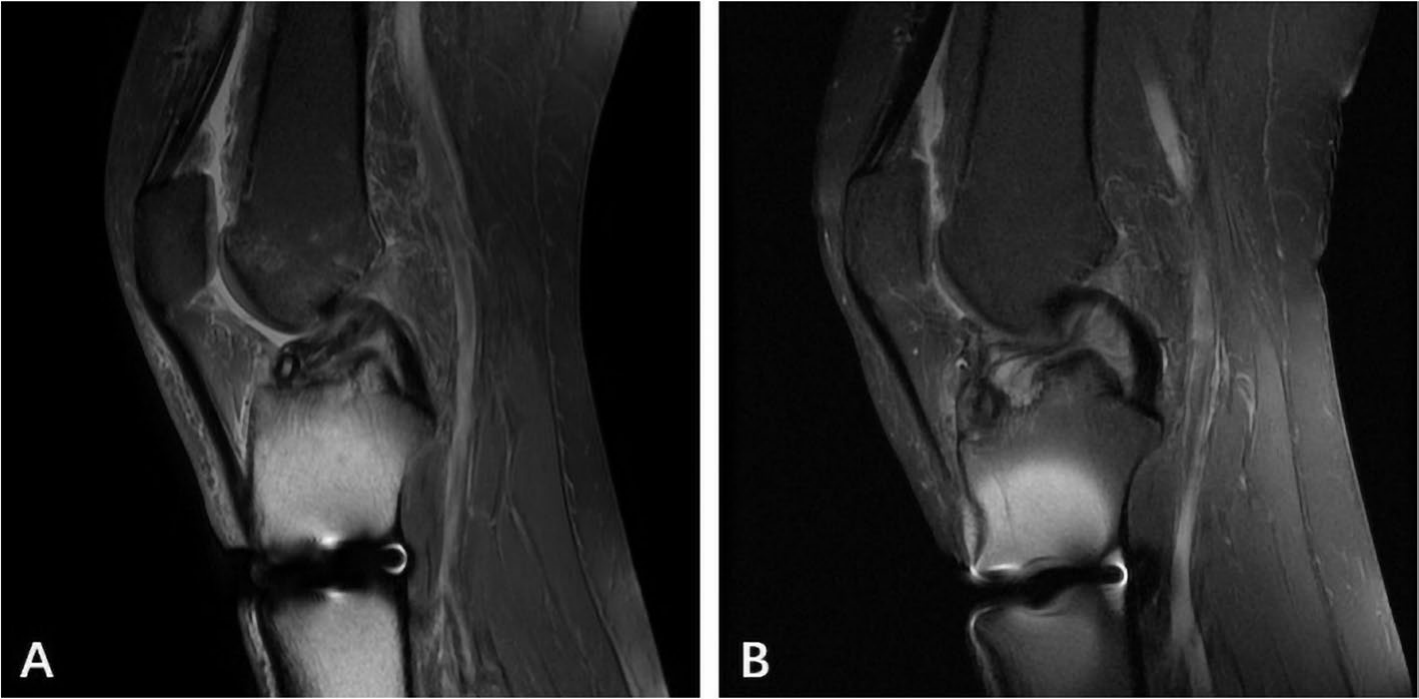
49-year-old female patients with ACL reconstruction: a one week after operation, MRI showed that the inner opening of tibial tunnel was anterior and the lateral tibial posterior slope (LTPS) was 14°; b half a year after operation, the continuity of ACL reconstruction was interrupted and fluid was accumulated at the internal opening of tibial tunnel
